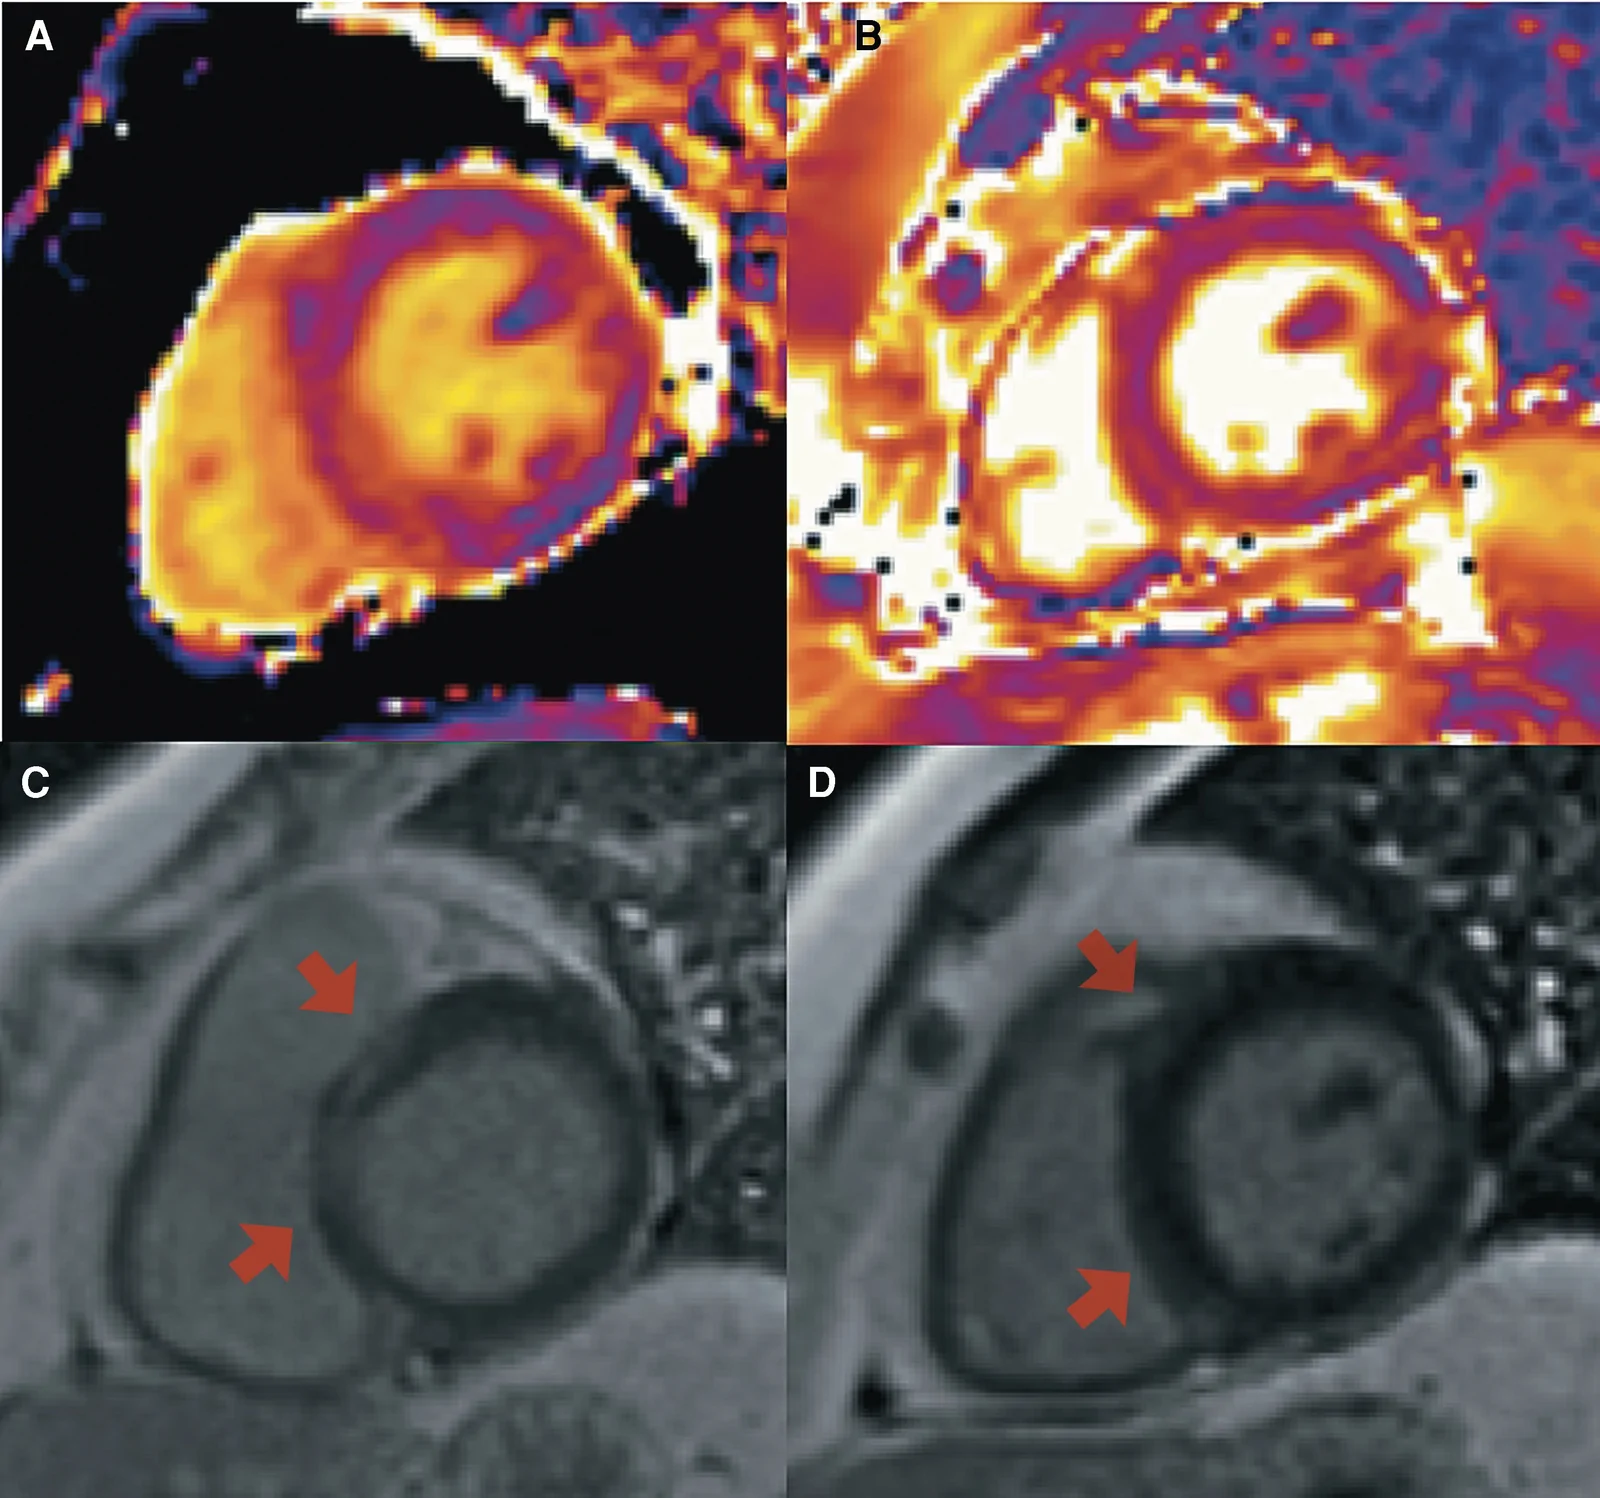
Cardiac magnetic resonance—non-ischemic late gadolinium enhancement. *(A)* T2-weighted turbo spin-echo sequences showing no evidence of myocardial edema. *(B)* Late gadolinium enhancement sequences demonstrating intramyocardial enhancement in the basal septal wall segment (arrows). *(C)* Quantitative myocardial T1 mapping showing evidence of interstitial fibrosis in the basal septal wall segment. *(D)* Quantitative myocardial T2 mapping showing no evidence of myocardial edema.
